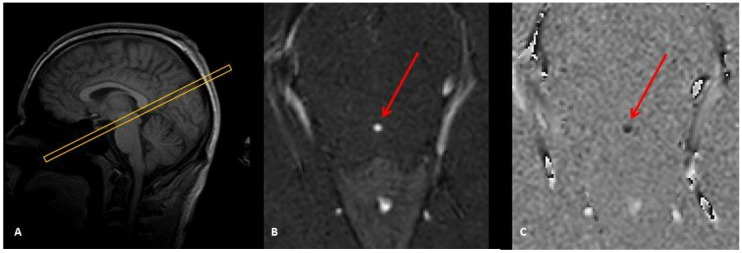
Sagittal image scout (A) to localize the aqueduct and to prescribe a perpendicular 2D Cine-phase MRI slice. This provides a magnitude image (B) suited to visualize and select the aqueduct (red arrow) and a phase-difference image to map the CSF flow (C). (B) and (C) represent one phase of the cardiac cycle.
